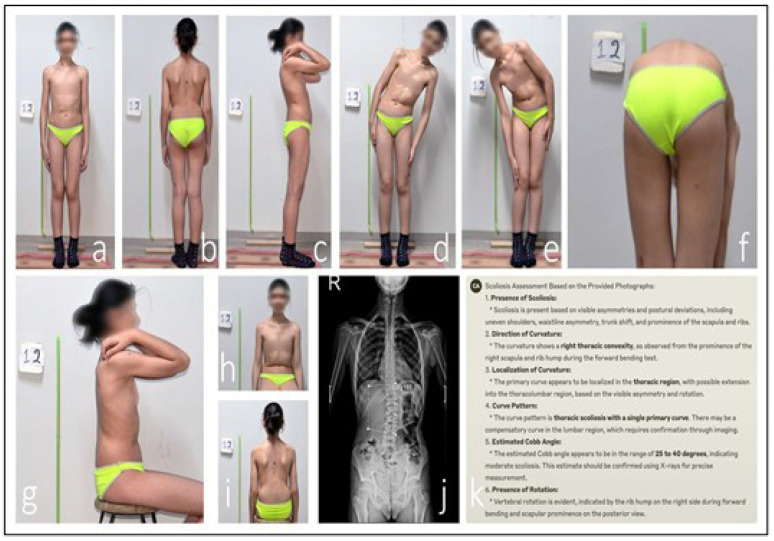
Discrepancy in Claude AI-assisted scoliosis assessment. An analysis of the patient’s clinical photographs by the Claude AI system identified a single structural right-convex thoracic curve with an estimated Cobb angle of 25–40° (a–h,k). This view demonstrates spinal alignment and scapular symmetry while seated, helping differentiate structural curves from postural deviations (i). Radiographic evaluation, however, measured a lumbar Cobb angle of 15° (j). This discrepancy indicates that Claude misclassified the primary curve location and significantly overestimated its magnitude.
